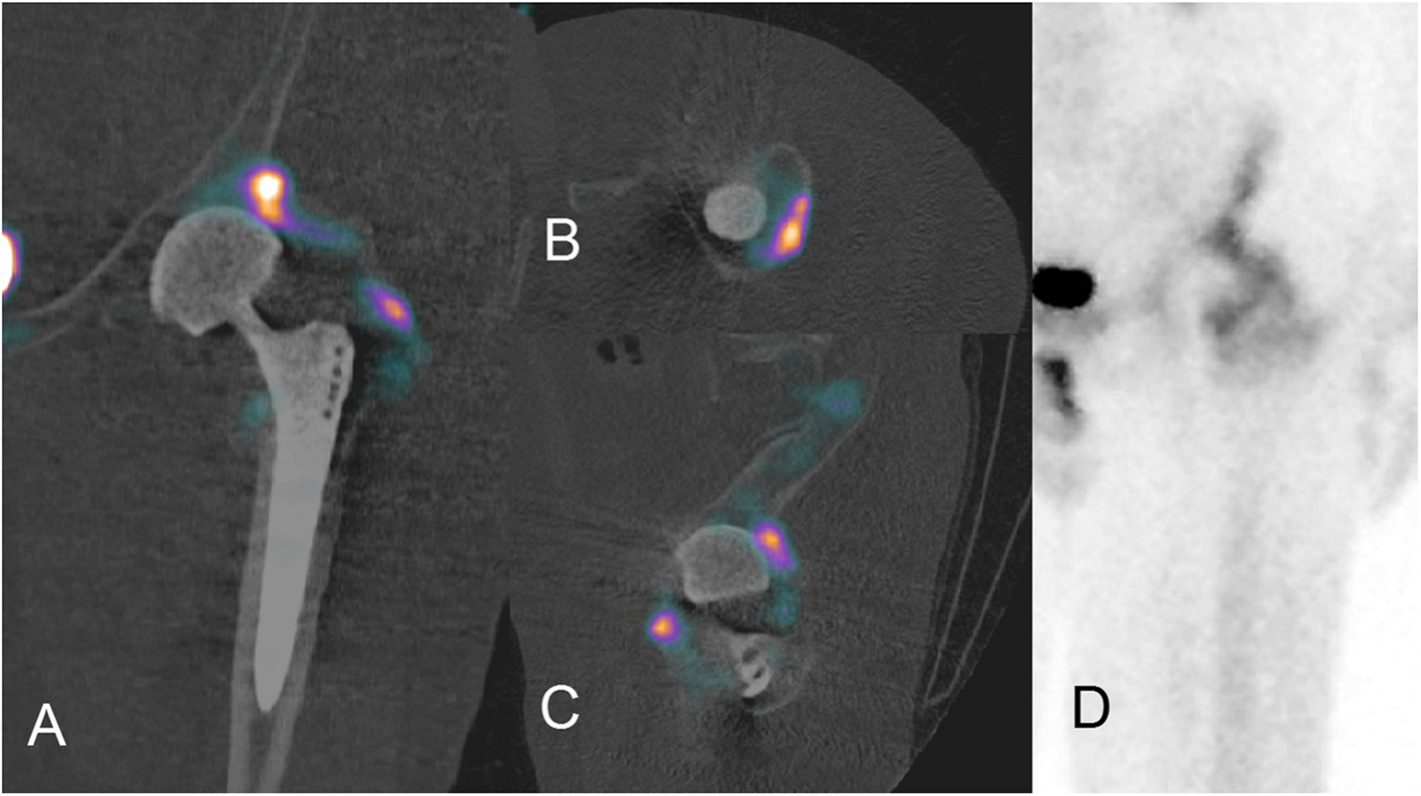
Differentiation between heterotopic ossifications and loosening of the acetabular component. 58-year old male with pain in the left hip that started several months after surgery. Using SPECT/CT, the increased uptake can be traced to heterotopic ossifications close to the acetabular component differentiating between loosening of the cup A)-C). D) shows the planar SPECT image of the left hip with increased acitivity around the cup that cannot clearly be assigned to a specific structure. After a subsequent scintigraphic examination proving inactivity of the ossifications, these were removed surgically.
